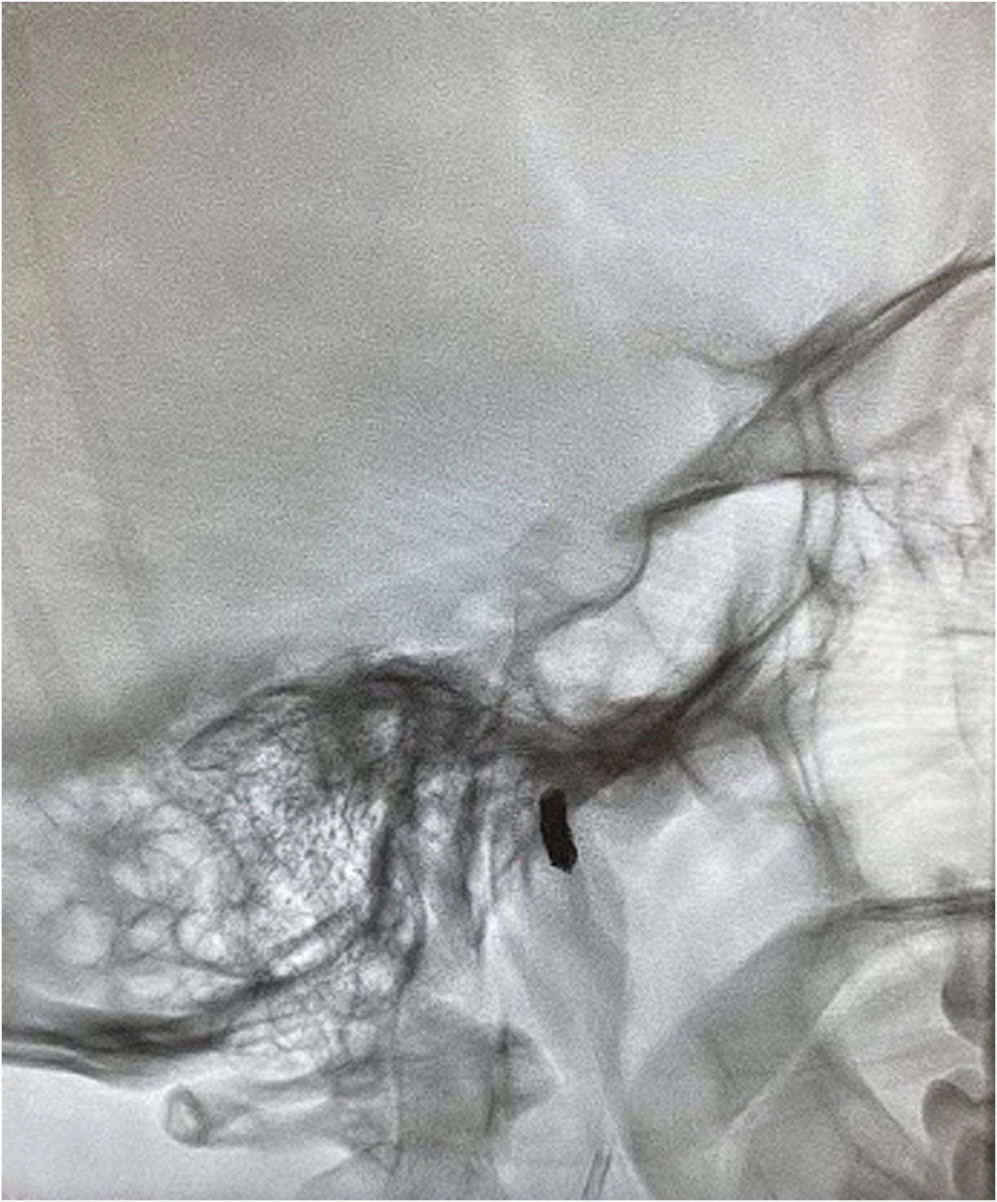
Plain film radiograph demonstrates embolization of the superficial temporal artery with coils
